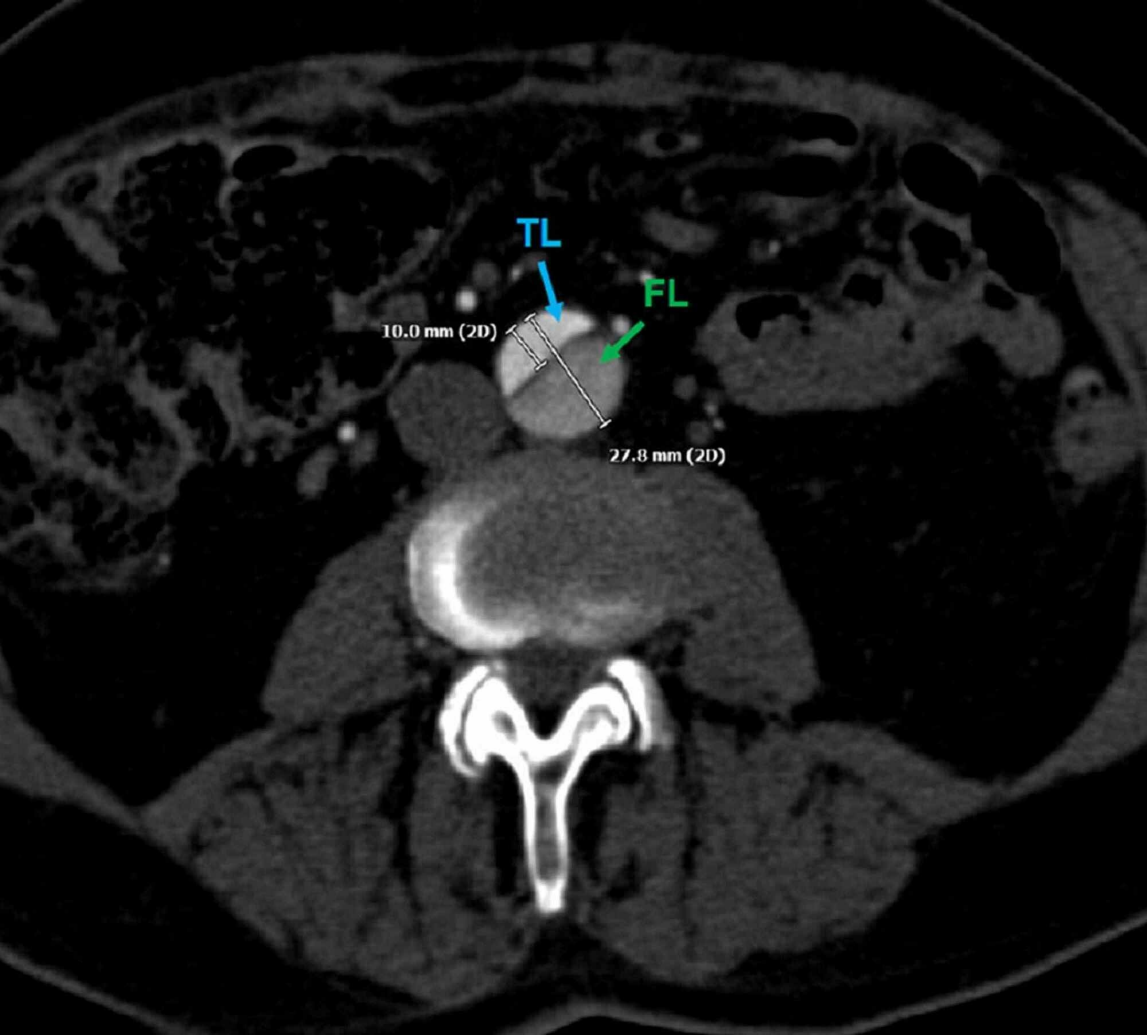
Axial Computed Tomography Angiography of a Pre-Procedural Stanford B Aortic Dissection A pre-procedural axial computed tomography angiogram shows the caliber sizes of the respective true (blue arrow) and false (green arrow) dissection lumens. The aortic diameter measures 27.8 millimeters in diameter at this level. The false lumen measures 17.8 millimeters and is noticeably larger than the true lumen, measuring 10 millimeters.
